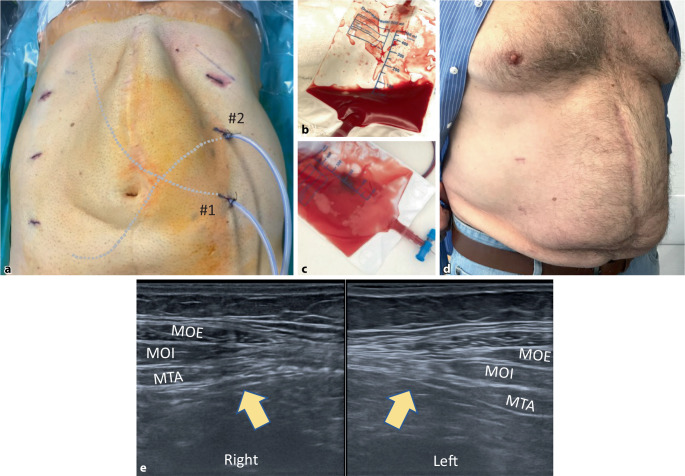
Postoperative course after robotic transversus abdominis release (r-TAR). a Final aspect after completion of surgery, showing the 3 accesses on each side as well as the two silicone drains (*#1* is located subphrenically, *#2* is located in the retropubic space); clearly visible is the subcutaneous bulge after endoscopic suture of the linea alba. b Bloody drainage secretion on the first postoperative day and c clear quality change of the drainage content to serous from the postoperative day 2 onwards. d Same patient as after 3 months. e Transverse ultrasound image of the linea semilunaris bilaterally (*yellow arrows*); 3 months after r‑TAR, there is neither retraction nor atrophy of the transversus abdominis. *MOE* external oblique; *MOI* internal oblique; *MTA* transversus abdominis
